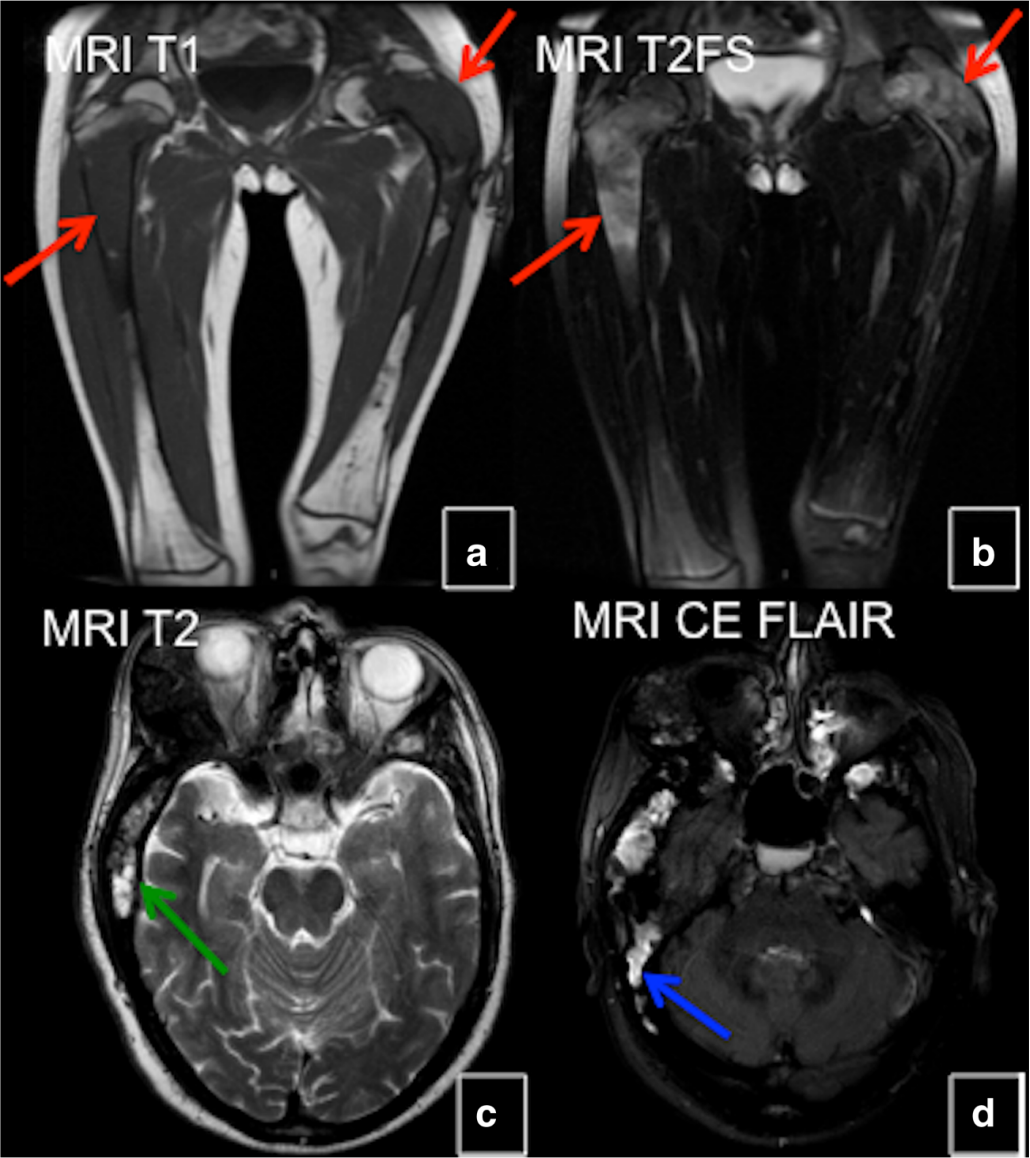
MRI in fibrous dysplasia (FD). a, b MRI typically shows sharply demarcated lesions with intermediate to low signal intensity on T1-weighted images (WI) and intermediate to high on T2-WI (*red arrow*). c Some FD lesions may also contain small cystic areas, which make the T2 signal bright (*green arrow*). d FD lesions usually show some degree of enhancement after contrast administration (*blue arrow*)
